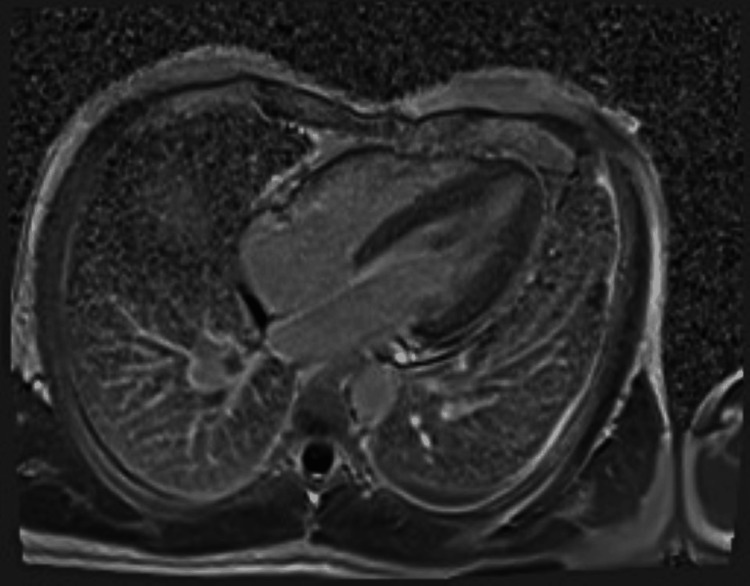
Cardiac MRI showing right atrial thrombus (delayed gadolinium)
